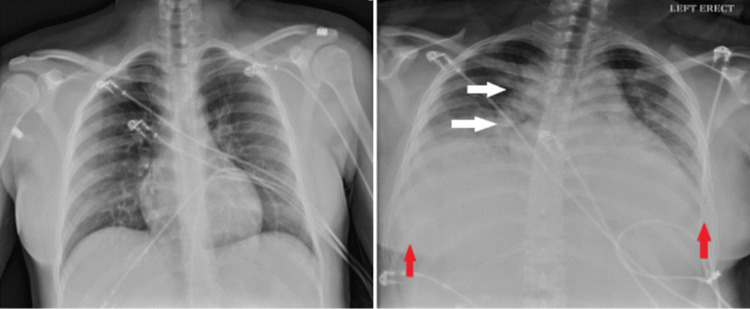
Normal chest X-ray on admission (left) and chest X-ray on day 2 (right) notable for pulmonary edema (white arrows) and bilateral pleural effusions (red arrows) with cardiomegaly
